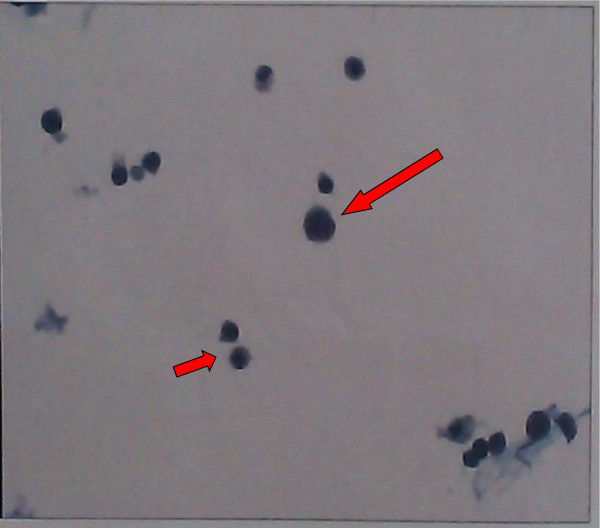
Scrotal ultrasound fine-needle aspiration cytology revealed giant cells with large nuclei and coarse chromatin (long arrow). Some intermediate-sized cells were noted (short arrow).
